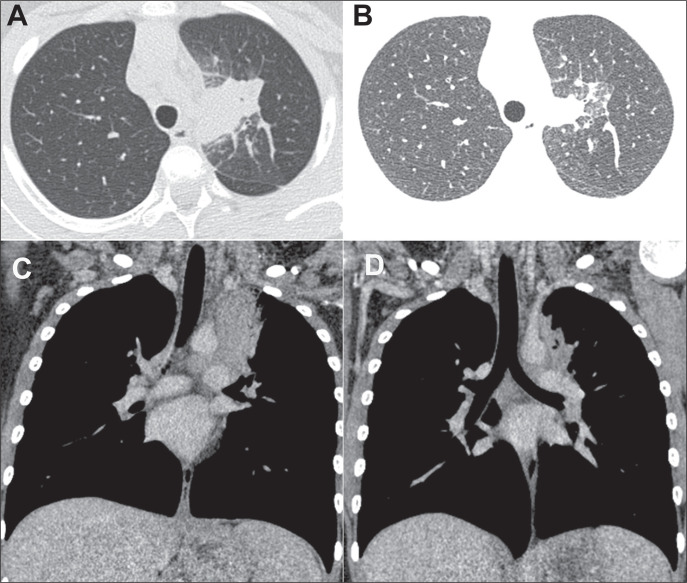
A 9-year-old girl. A,B: CT scans showing a mass in the upper left
